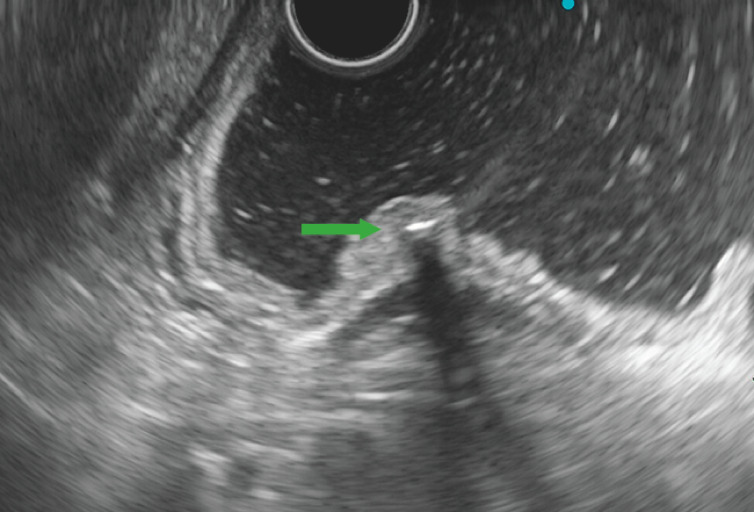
Endoscopic ultrasonography image showing a hyperechoic lesion in the gastric submucosa.
